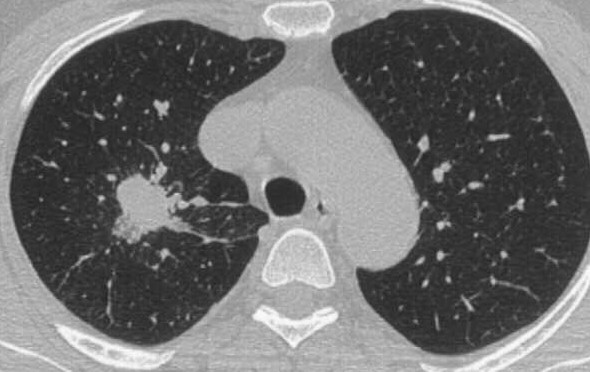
Preoperative CT scans. The preoperative CT scans clearly demonstrate the main tumor in the upper lobe of the right lung.
